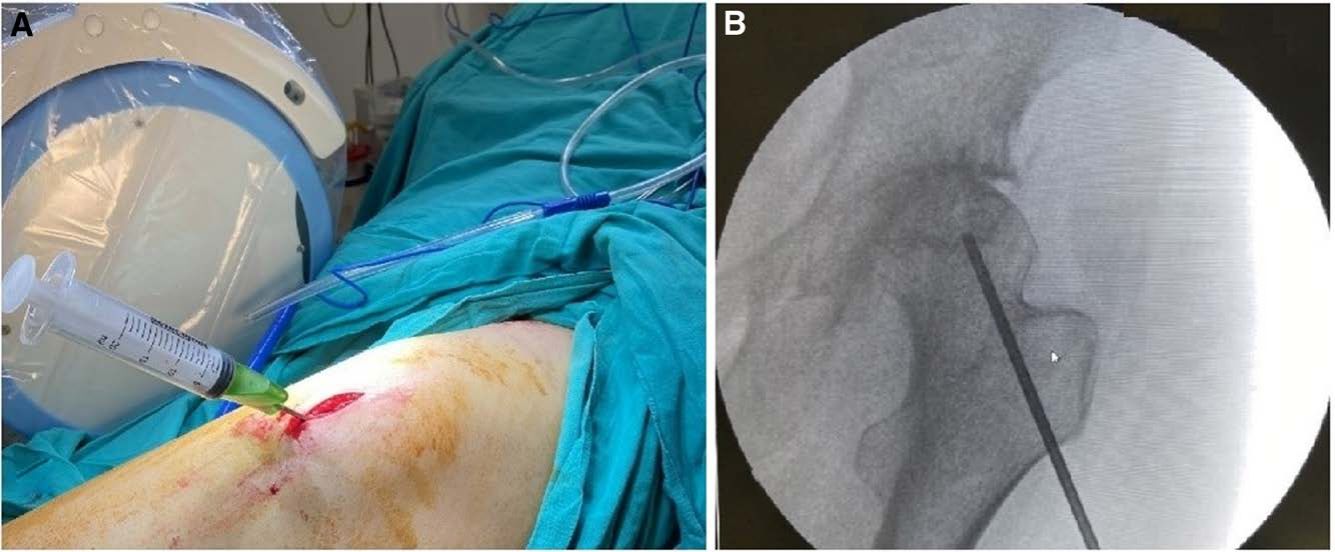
(A) Intraoperative surgical view of the bone marrow aspirate injection. (B) Anteroposterior X-ray view of the bone marrow aspirate injection.
